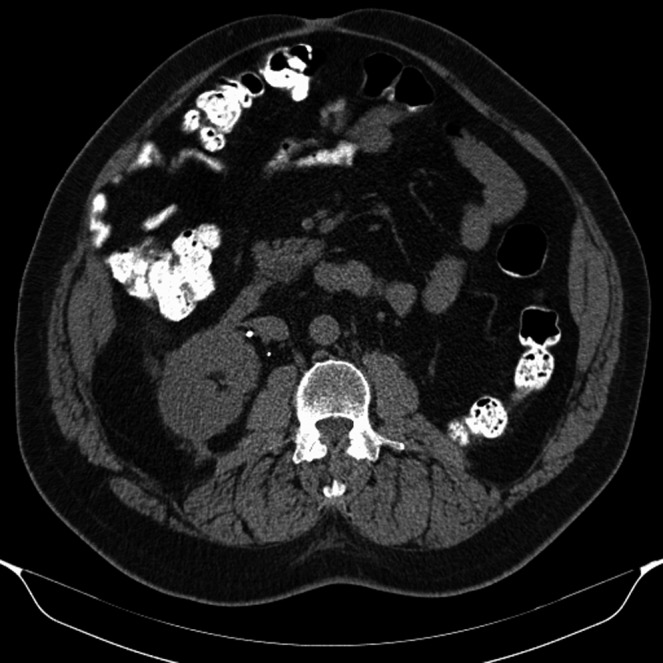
CT scan obtained 1 year after second resection. No evidence of recurrent disease. The right kidney has returned to its natural morphology and kidney function remains adequate.
